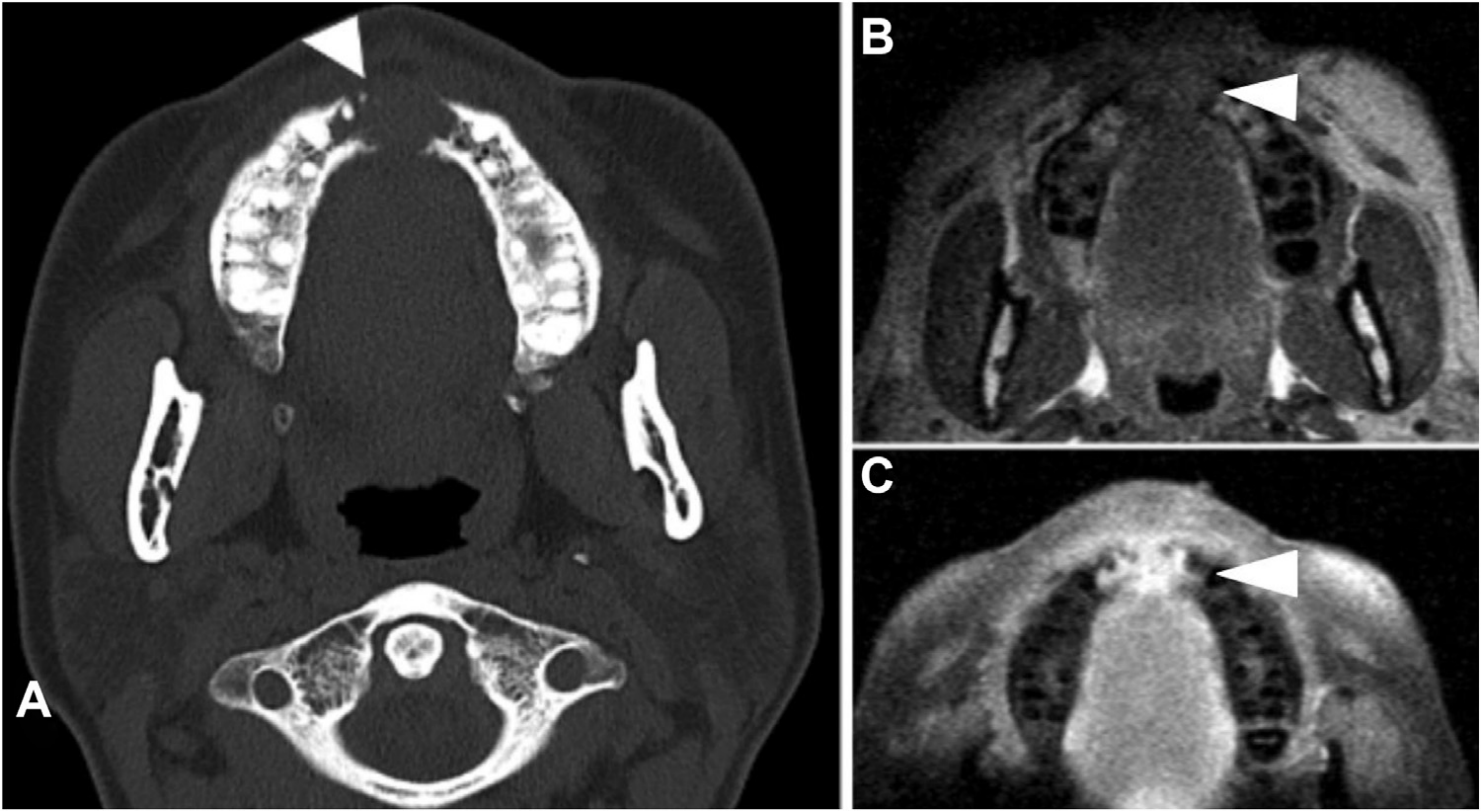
(A) Axial CT of the sinus without contrast demonstrates an erosive mass within the anterior aspect of the lower maxilla (white arrowhead). (B and C) Pre and Postcontrast Axial T1 images show an enhancing T1 isointense mass in the anterior lower maxilla (white arrowheads).
